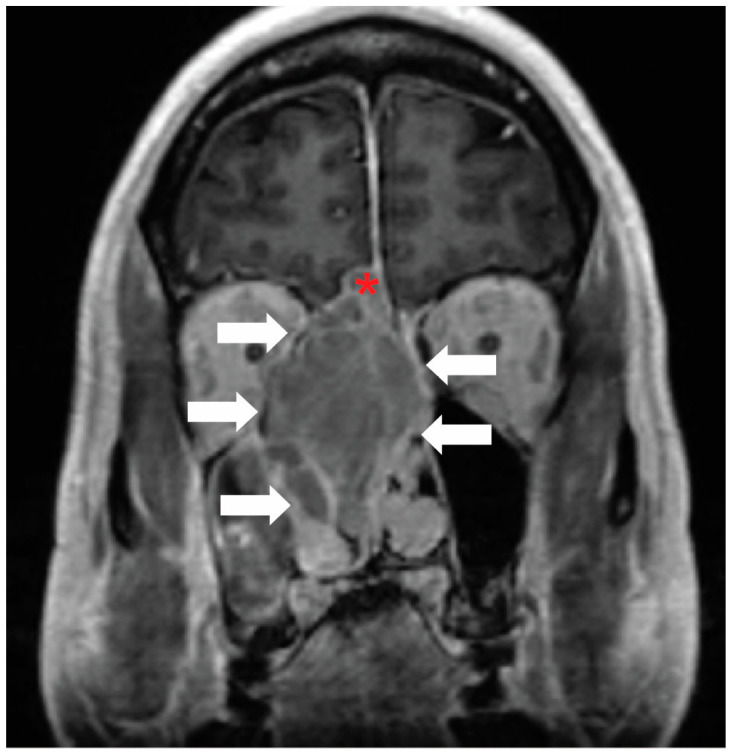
Classical tumor visualization: Contrast-enhanced coronal MRI of the face demonstrates an esthesioneuroblastoma in the right ethmoid air cells (white arrows). There is also intracranial extension (red asterisk). Image and annotations are from the authors.
